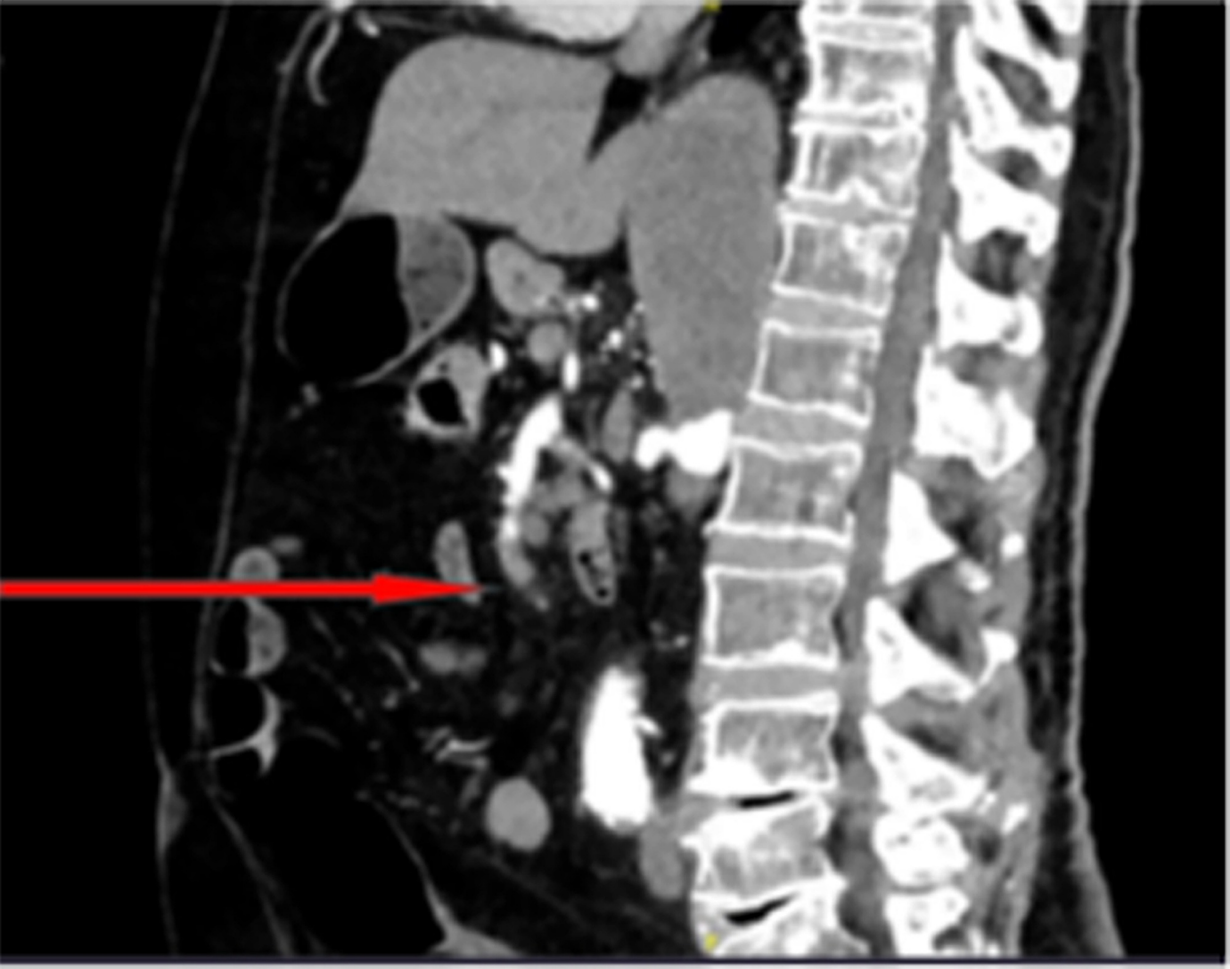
CT with contrast showing a distal occlusion of SMA. SMA, superior mesenteric artery
